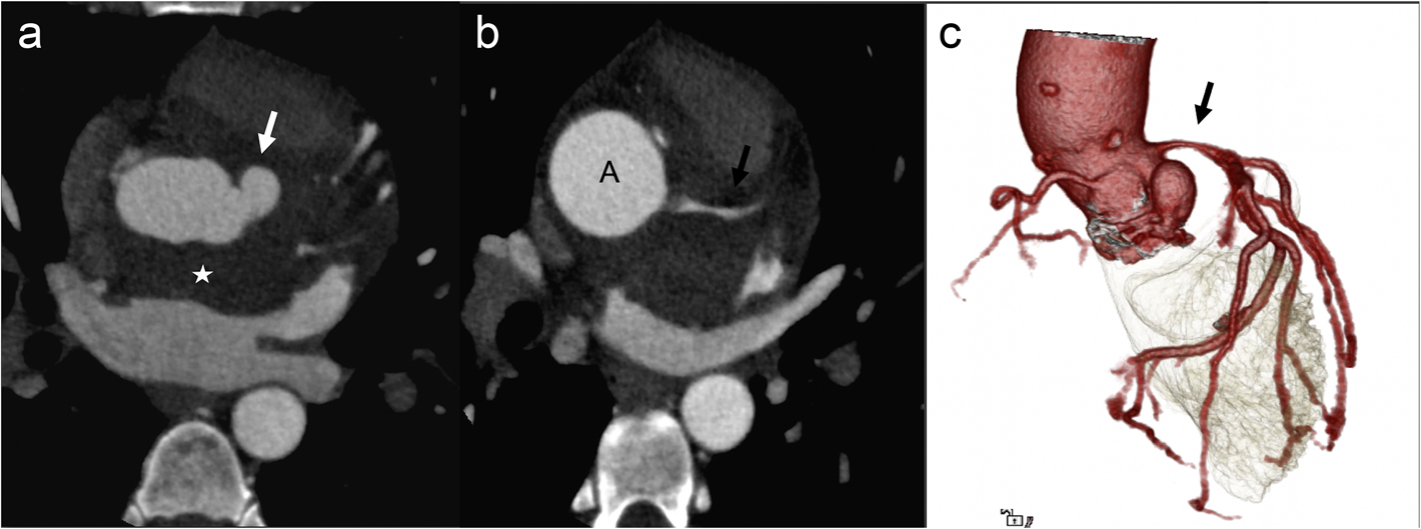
A 33-year-old male with a history of intravenous drug abuse with infectious endocarditis status post aortic valve replacement, presenting with fever. Axial (a and b) and volume-rendered (c) CTA images showing soft tissue (star) between the aortic root and the left atrium consistent with a paraaortic abscess. A pseudoaneurysm (white arrow) is also seen, and there is a mass effect on the left main coronary artery (black arrow) with moderate narrowing
